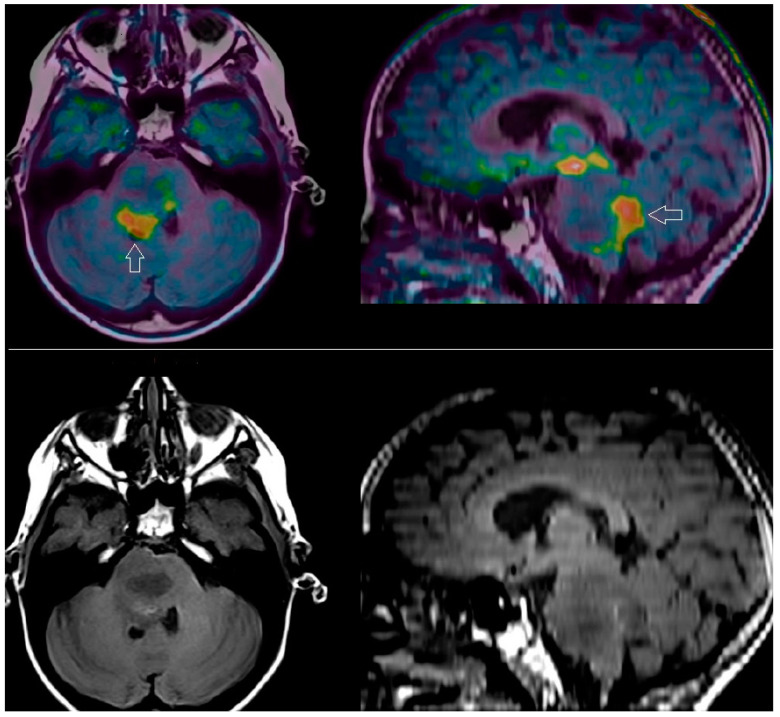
An 8-year-old female, diagnosed with diffuse intrinsic pontine glioma (DIPG), previously submitted to chemo-immunotherapy and radiotherapy. Amino-acid PET/MRI (post-processing co-registration) with [^18^F]-DOPA (upper row), carried out 8 months after RT demonstrated a rim of residual viable tumor tissue (arrow), evident on the axial (left) and sagittal (right) slices, within the posterior portion of the hypointense area revealed by MRI (lower row; axial on the left, sagittal on the right).
